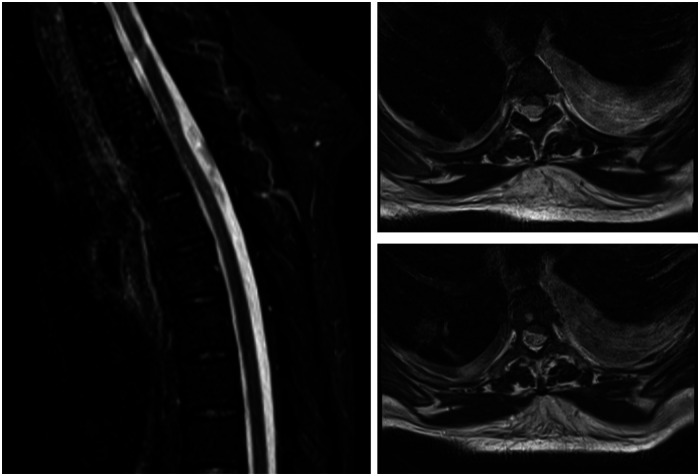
A sagittal T2 weighted and corresponding axial cross sections at two levels. MRI shows an iso- to hypointense possible intradural extramedullary lesion extending from thoracic level two to thoracic level five, which compressed the spinal cord and displaced it anteriorly.
